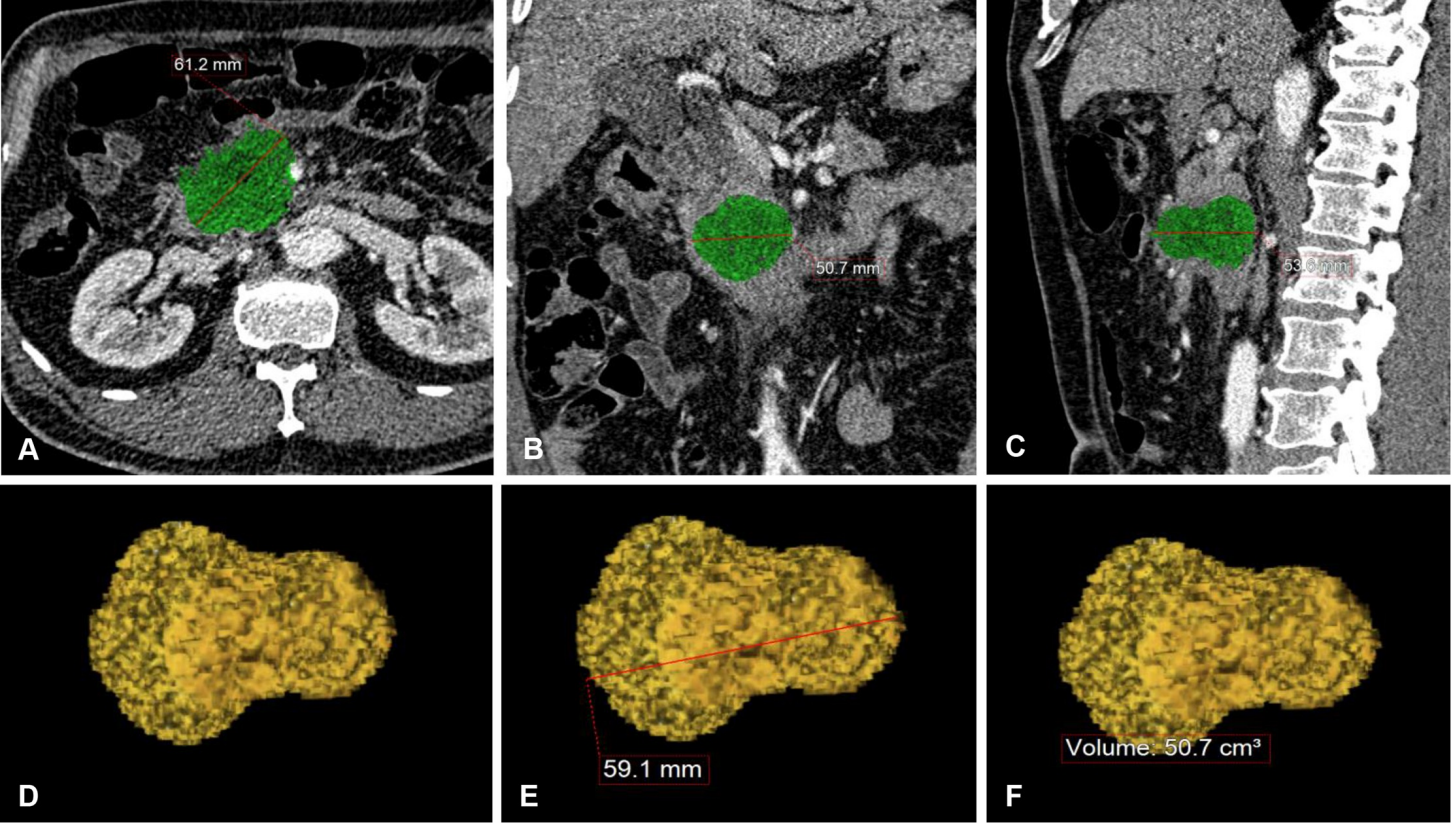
Measuring the diameter and volume of pancreatic cancer in multi-planes and three-dimensional (3D) computed tomography (CT) images. The maximal tumor diameters were measured in the axial (A), coronal (B), and sagittal (C) planes using CT images. A 3D CT image for each tumor was obtained through a semi-automated segmentation method (D), and maximal diameter on 3D images (E) and cancer volume (F) were measured.
